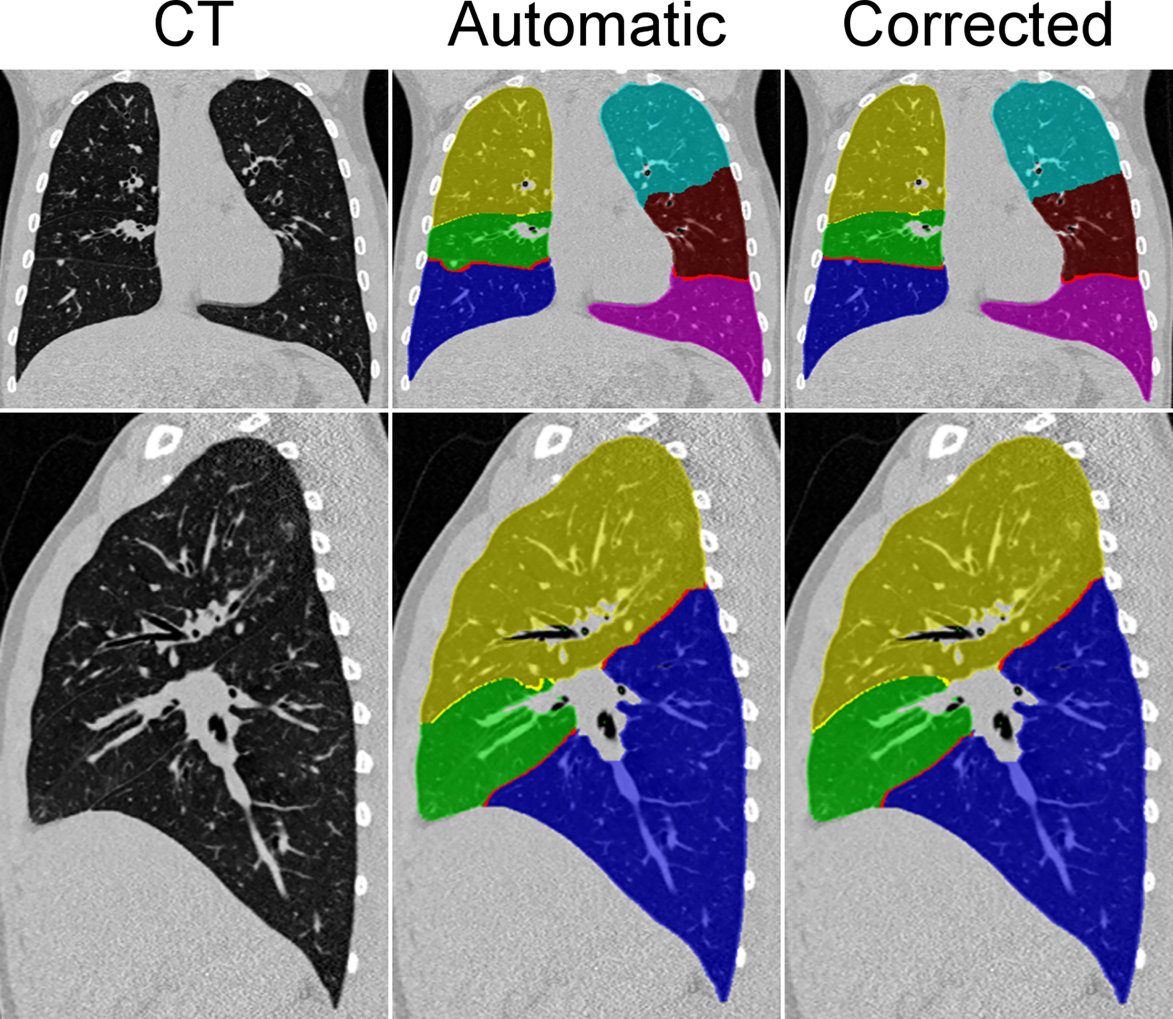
Representative example of the manual correction process of the automatically segmented lung lobes. Coronal as well as sagittal views of the right lung are shown for the original CT data and the results after automatic lobe segmentation (“automatic”) in this CF patient. The course of the fissures was already satisfactorily detected by the software. Manual correction lead to a smoother delineation but did not substantially change the position of the margins of the lobes. Lobes are indicated as follows: yellow = right superior lobe, green = middle lobe, blue = right inferior lobe, light blue = left superior lobe, maroon = lingual, purple magenta = left inferior lobe.
